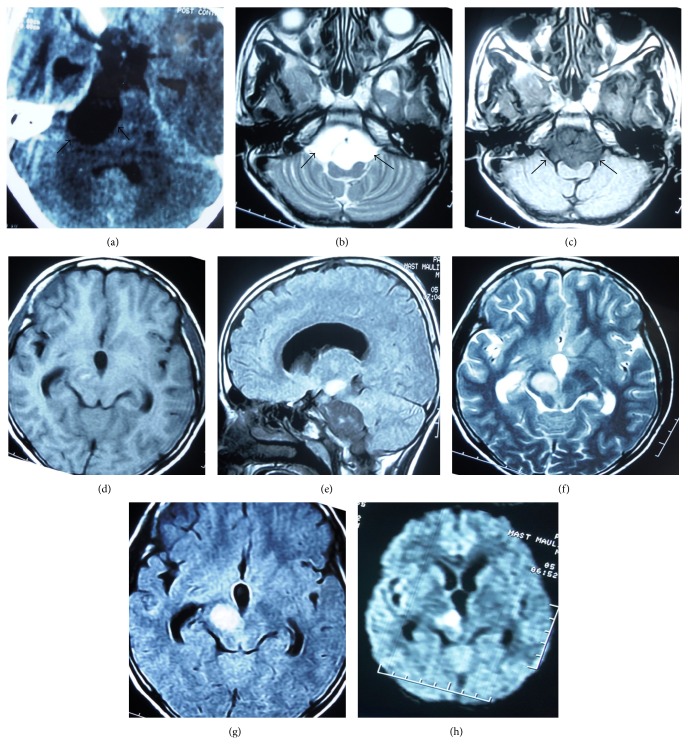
(a) Axial image of plain computed tomography (CT) showing hypodense lesion in right cerebellopontine angle and prepontine cistern with compression of the brainstem. Axial T2 (b) and T1 (c) weighted MR images showing the lesion to be hypointense on T1 and hyperintense on T2 sequence (black arrows outline the tumour). (d) and (e) are T1 weighted axial and sagittal images. (f), (g), and (h) are axial T2, FLAIR, and diffusion weighted images, respectively, revealing another lesion of hyperintensity and restricted diffusion in right half of midbrain.
